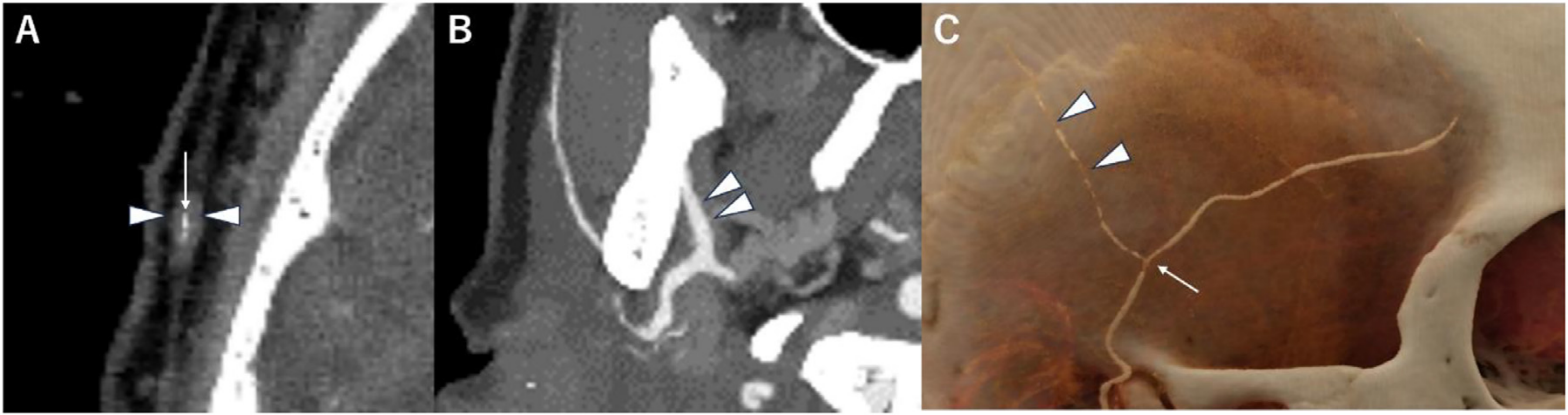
(A) CT angiography at the bifurcation of the frontal and parietal branches of the right superficial temporal artery shows a poorly enhancing thickened wall (arrowheads) with an enhancing luminal stenosis (arrow). (B) The thin-slab maximum intensity projection image at the maxillary artery shows no stenosis (arrowheads). (C) The cinematic rendering image depicts multiple stenoses of the parietal branch (arrowheads) and the bifurcation of the frontal and parietal superficial temporal artery branches(arrow).
